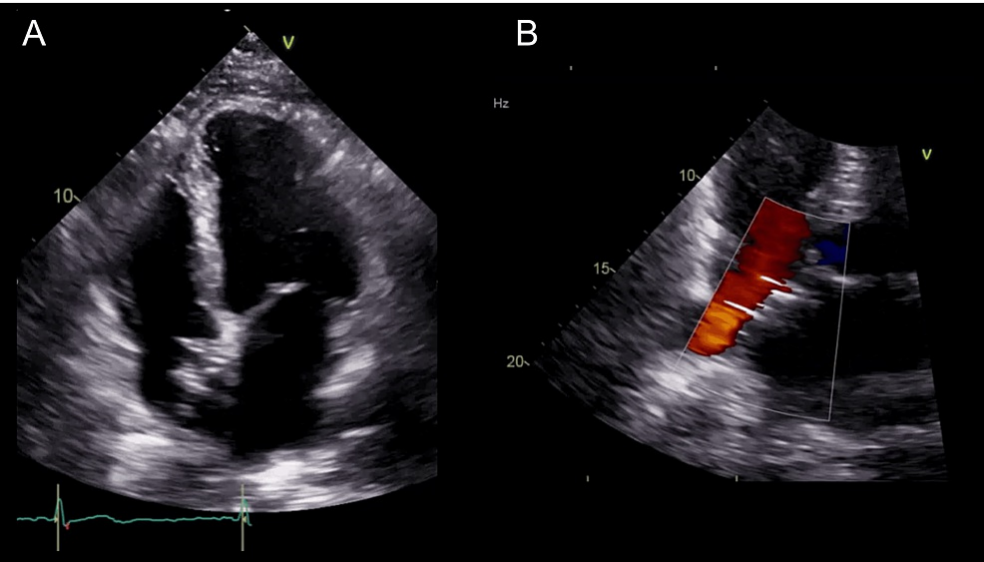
Postoperative TEE imaging (A) Postoperatively, there was no dislodgement of the 44-mm GCA. (B) No residual shunt after placement of the 44-mm GCA. GCA, GORE® Cardioform ASD Occluder; TEE, transesophageal echocardiography
